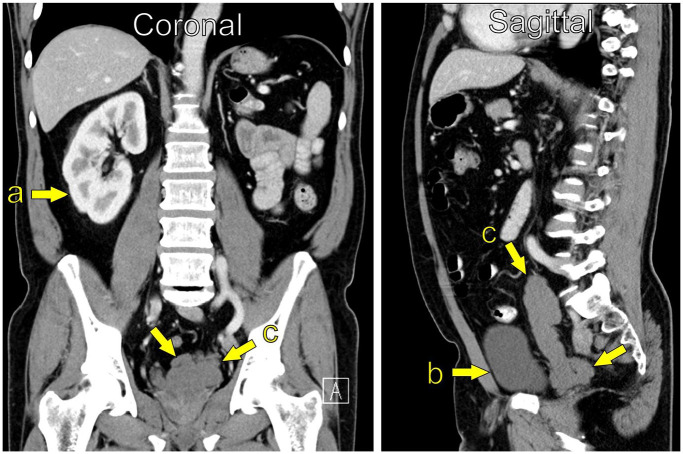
CT Abdomen/pelvis (Coronal and Sagittal reconstruction); Siemens Definition AS (Siemens Healthineers); unknown i.v. contrast-agent, unknown injection volume and injection rate. (a) Single kidney right side, (b) Bladder, (c) Cystic enlargement of the seminal vesicle, measuring 3.4 × 3.0 × 12.2 cm (ca. 62 mL).
